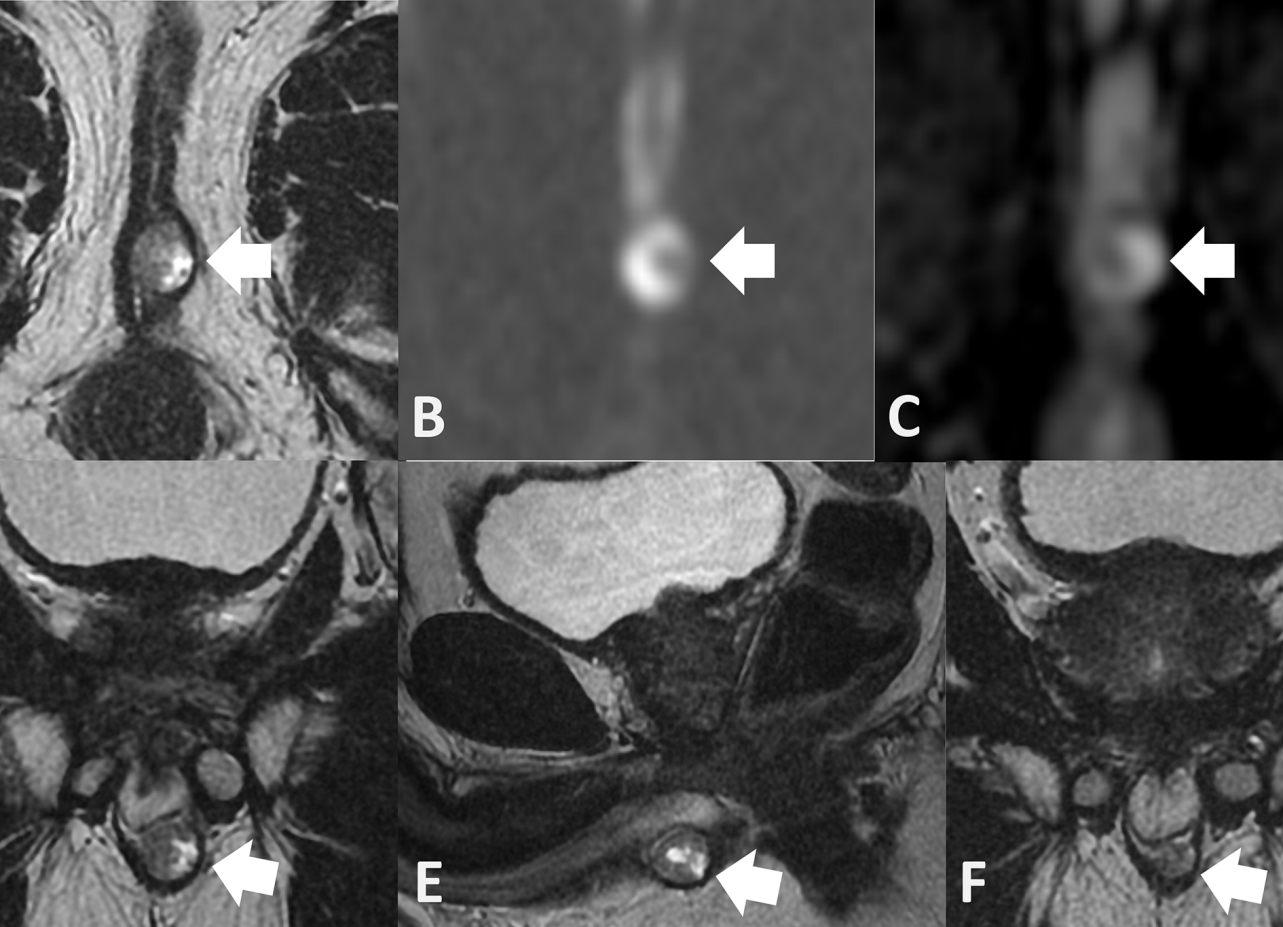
Axial *T*_2_ weigthed (A) DWI (B) and ADC map (C) shows a 15 mm mixed SI lesion (arrow) which is of high SI on the b-1400 diffusion scan and corresponding low SI on the ADC map indicating diffusion restriction. The lesion lies juxtaposed to the penile bulb and deep to the bulbocavernosus muscle on the coronal (D) and sagittal (E) scans. In retrospect, the lesion was present on the coronal *T*_2_ weighted MRI (F) performed 15 months earlier and is smaller (arrow). ADC, apparent diffusion coefficient; DWI, diffusion weighted imaging; SI, signal intensity.
